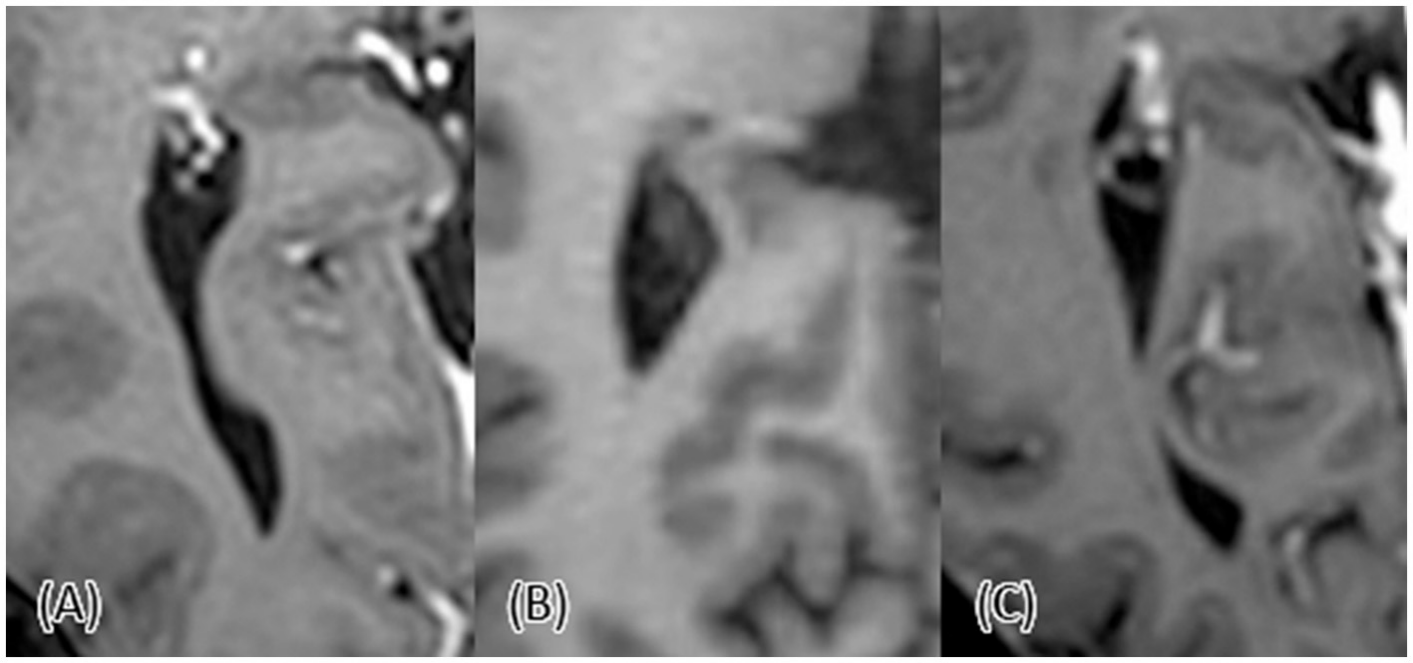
T1-weighted axial view of the posterior horn. (A) The posterior horn is open. (B) The posterior horn is closed. (C) The posterior horn is constricted with a tandem appearance.
